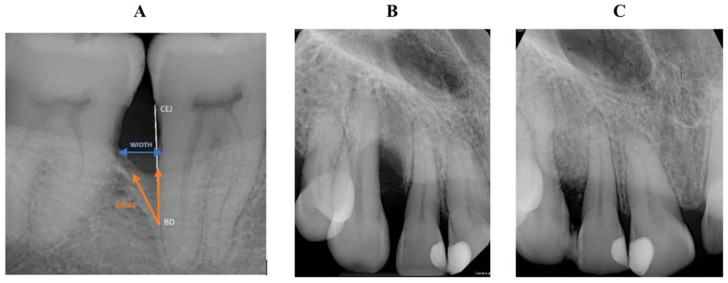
(A) Radiographical measurement parameters, showing cemento-enamel junction (CEJ), the width (WIDTH); the base (BD), and the angulation (ANGLE) of the periodontal defect; (B) Preoperative radiography showing the one-wall bony defect (C) postoperative radiography taken after six months. Radiographically, no periodontal defect can be detected.
